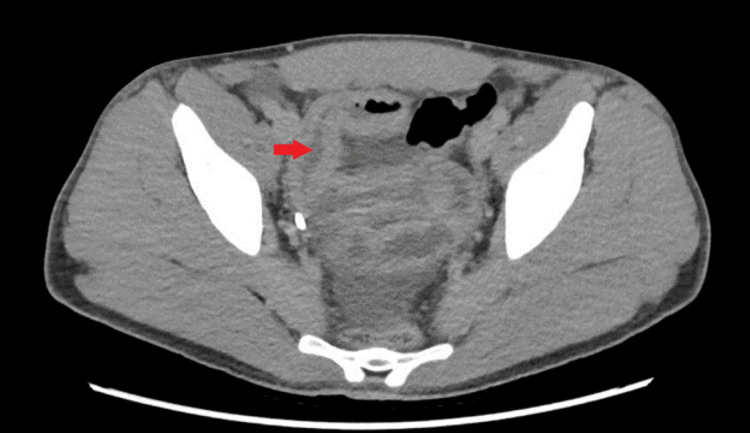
CT of the abdomen/pelvis showing inflammation of the terminal ileum Arrow indicates inflammation/thickened bowel wall of the terminal ileum/colon
